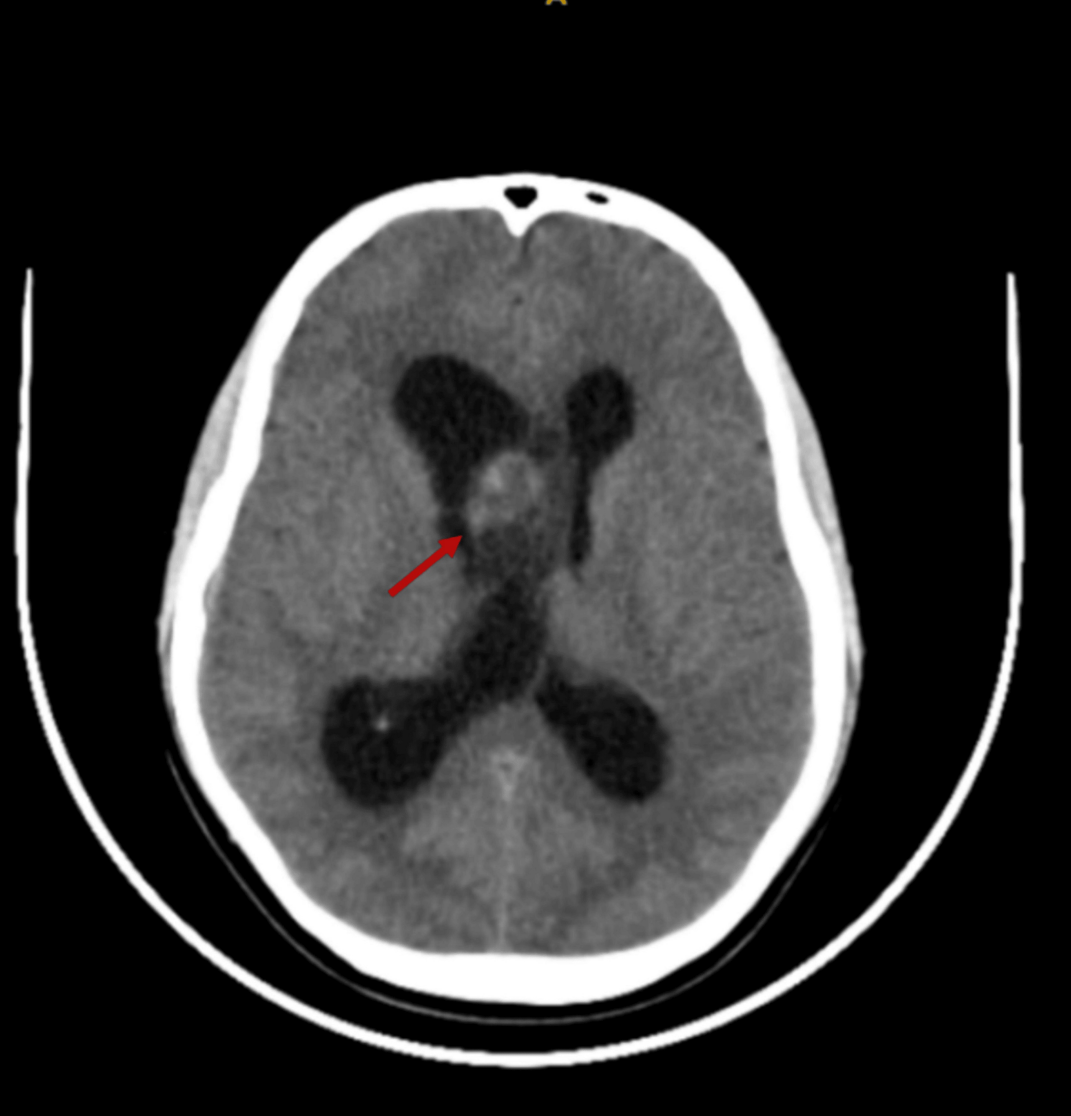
Contrast-enhanced computed tomography image, showing a well-defined heterogeneously enhancing solid cystic lesion with few nonenhancing hypodense along with few calcific foci within the foramen of Monro region (red arrow)
